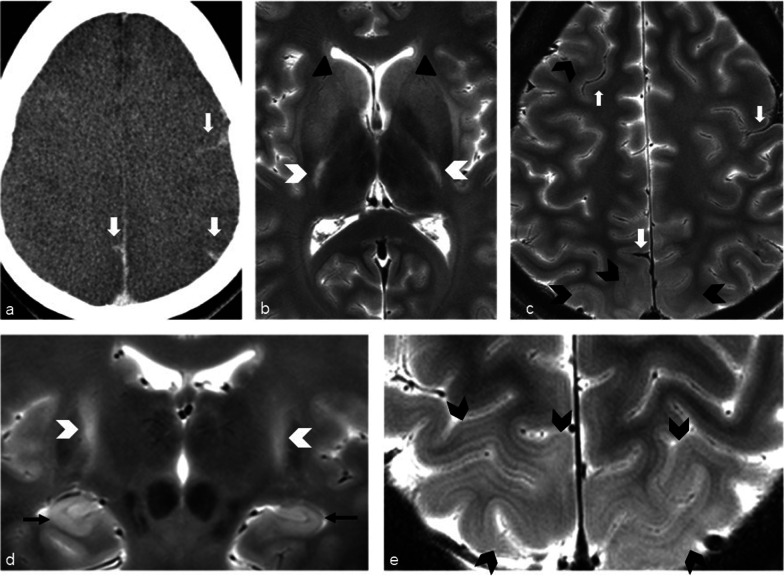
Patient 2. Bilateral subarachnoid hemorrhages (white arrows) in frontal and parietal sulci on CT (a) and axial T2 (c), signal abnormalities in the corticospinal tracts (white arrowheads b, d), bilateral basal ganglia (b—black arrowheads), hippocampi (d—black arrows), bilateral cortical/subcortical parietal lobes, and more subtle in the right frontal lobe (c, e—black arrowheads)
